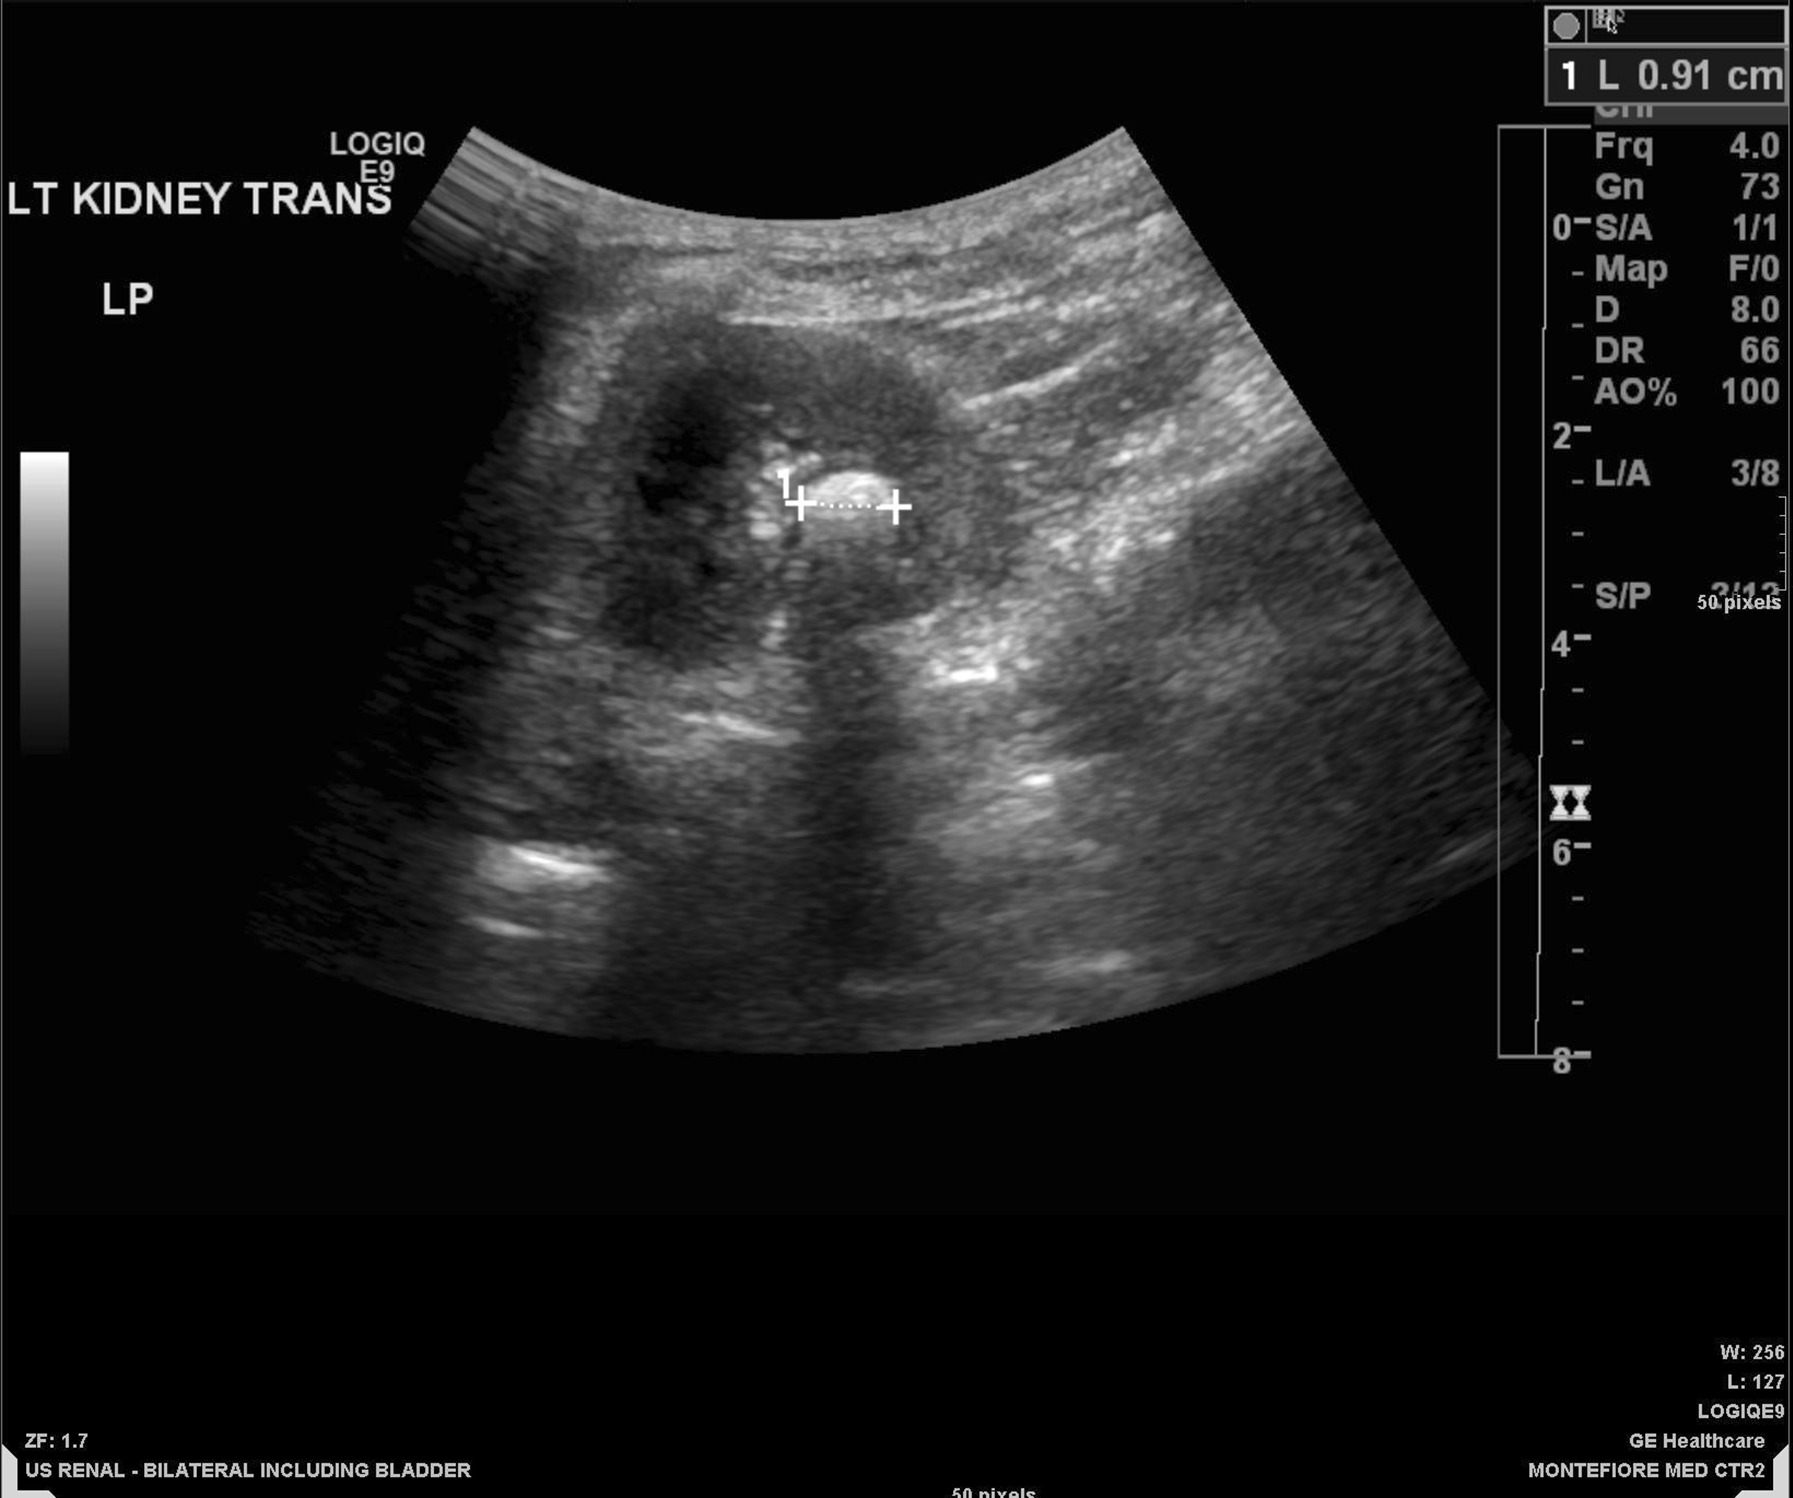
In vivo ultrasound of xanthine urinary stone: stone is echogenic with posterior acoustic shadowing
